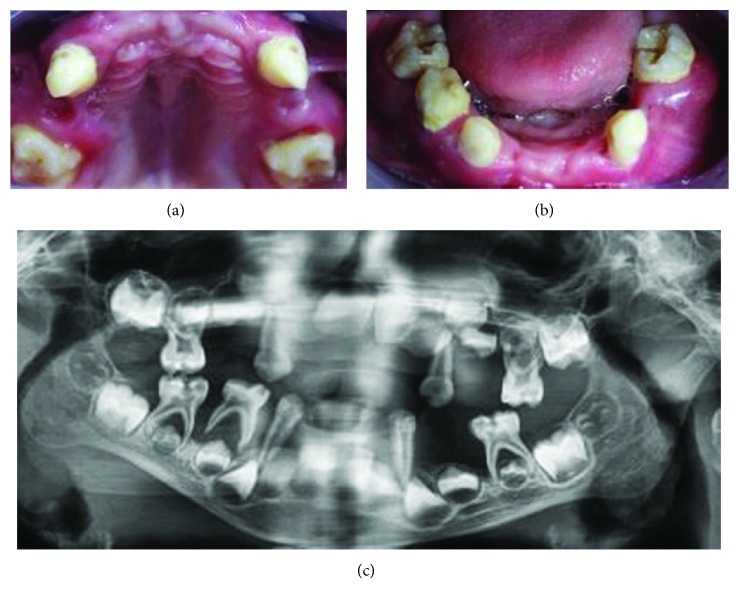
(a and b) Intraoral maxillary and mandibular aspects before multiple dental extractions. (c) Panoramic radiograph before extractions.
